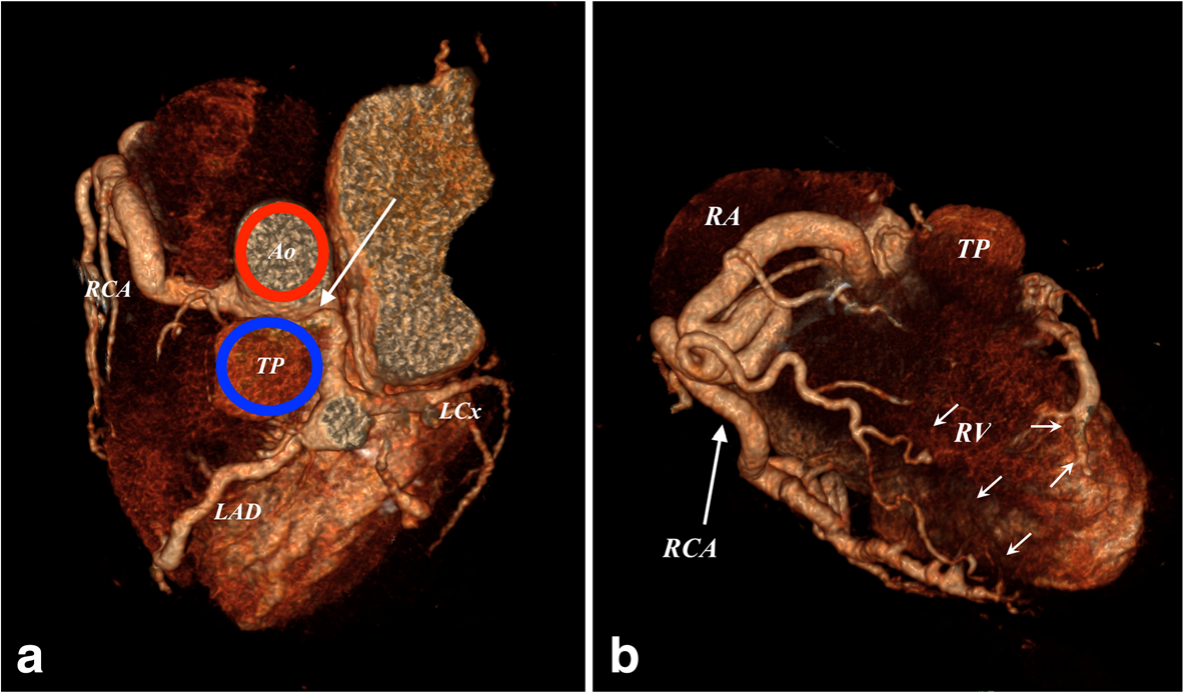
a and b Three-dimensional volume-rendered computed tomographic angiograms showing the anomalous origin of the left coronary artery from the pulmonary artery syndrome (*arrow* in a). Dilated intercoronary arteries (*short arrows* in b) can be seen along the epicardial surface, representing collateral pathways between the RCA and the left coronary artery. *Ao* Aorta, *LAD* Left coronary artery, *LCx* Left circumflex coronary artery, *RA* Right atrium, *RCA* Right coronary artery, *RV* Right ventricle, *TP* Pulmonary trunk
